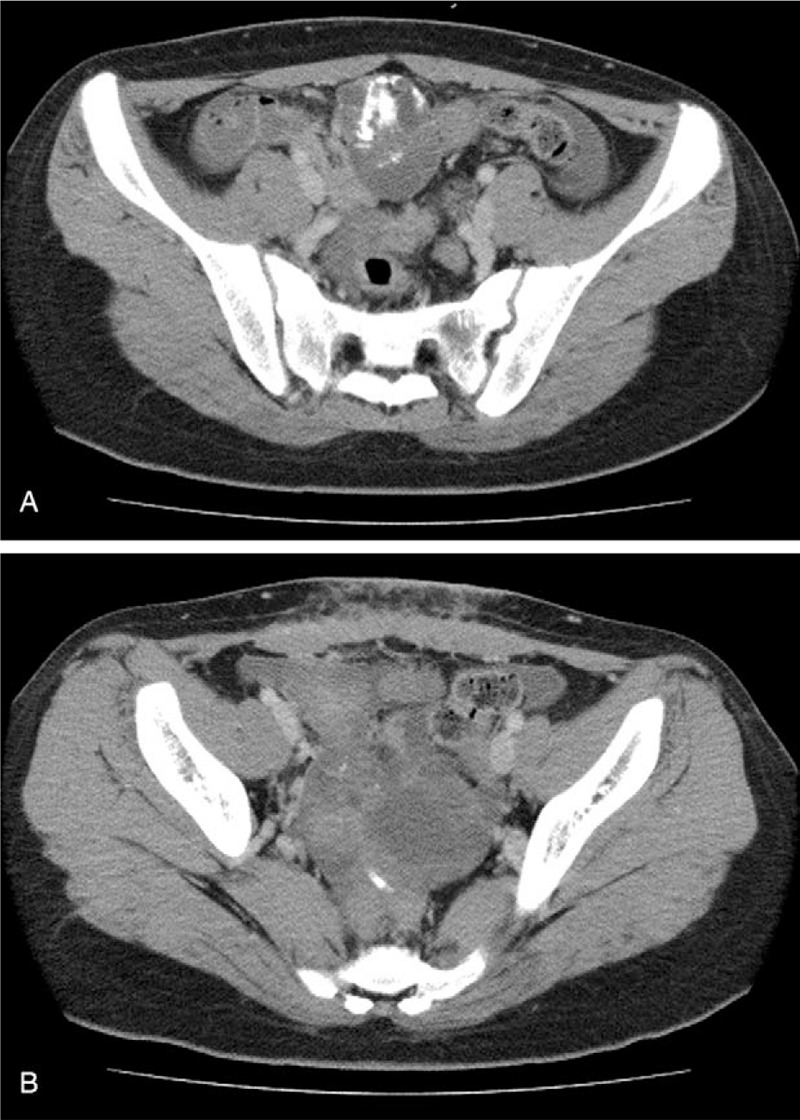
Abdominal computed tomography findings from 4 months after the first operation revealed multiple multinodular tumors containing calcified and fatty tissues (A). Invasion or lymph node swelling were not detected. Small amounts of ascites were observed around the liver and in the pelvic cavity (B).
